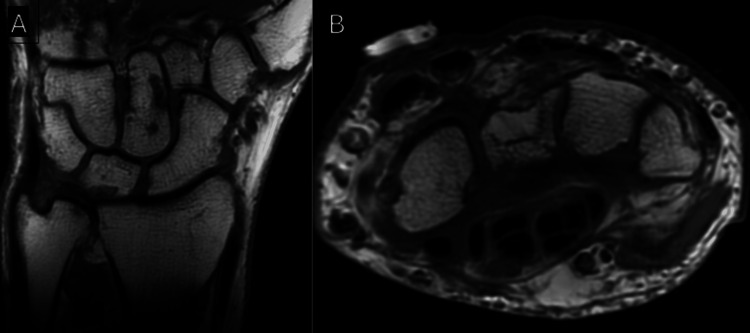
(A) Coronal and (B) axial MRI of the patient's carpus, 1 year post-resection, demonstrating no evidence of recurrence of nodular fasciitis.
